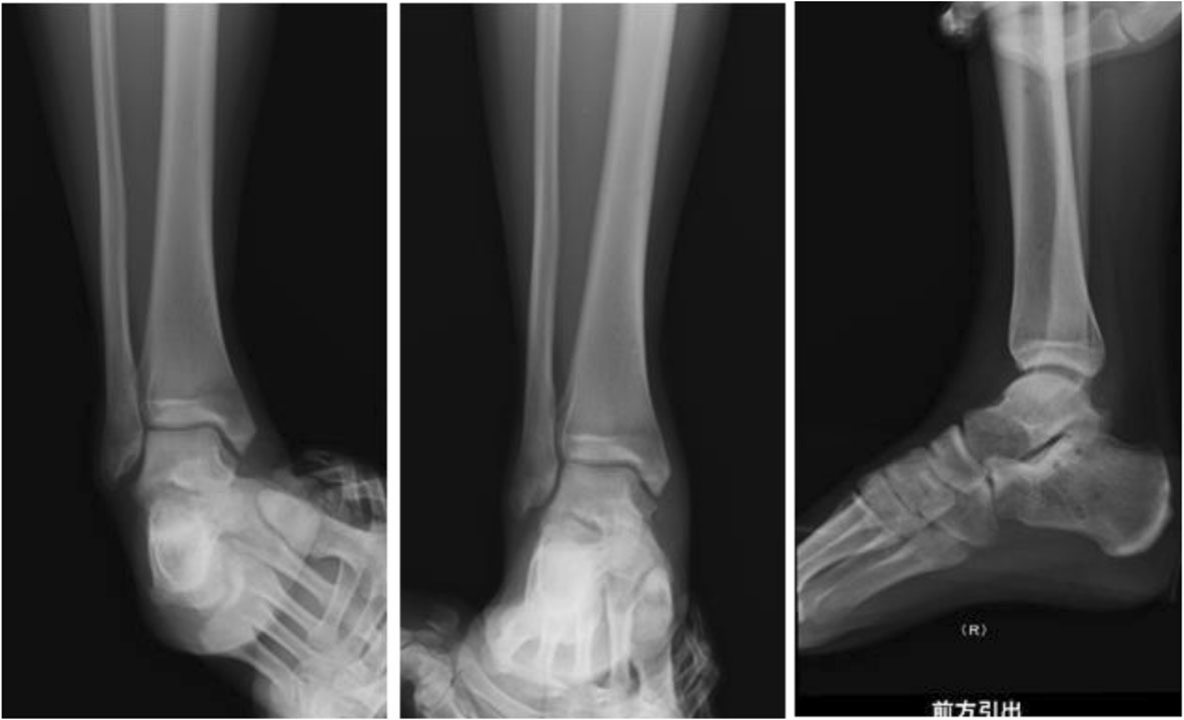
The varus and valgus stress views and anterior drawer view in X-ray 4 months postoperatively. Adequate stability of the ankle joint was observed
